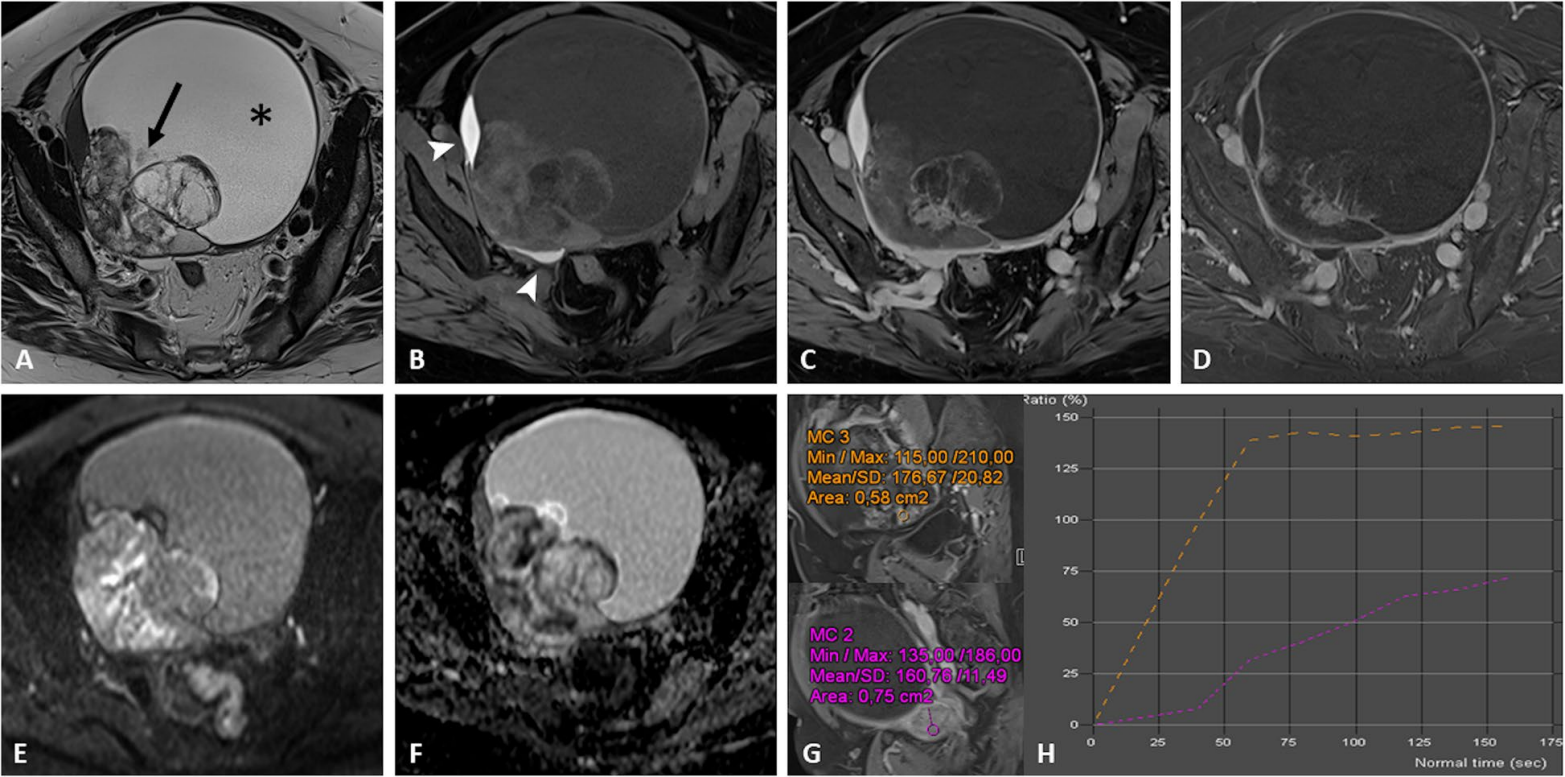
Clear cell carcinoma on right ovarian endometrioma in a 66-year-old woman with a palpable abdominal mass. Axial T2-weighted image (a) shows a mixed cystic (asterisk) of the right ovary containing a large peripheral solid portion (arrow). Hemorrhagic component (arrowhead) corresponding to endometriomas are displayed on axial fat-suppressed T1-weighted image (b). Enhancement of the solid portions is seen on axial contrast-enhanced fat-suppressed T1-weight image (c) and corresponding subtraction image (d). Diffusion-weighted imaging (e, f) shows restricted diffusion of neoplastic portions. Dynamic contrast enhancement analysis (g high *b* value and h ADC map) shows a type 3 curve regarding the solid portions (orange curve) located above the myometrial reference curve (pink curve), consistent with malignancy
